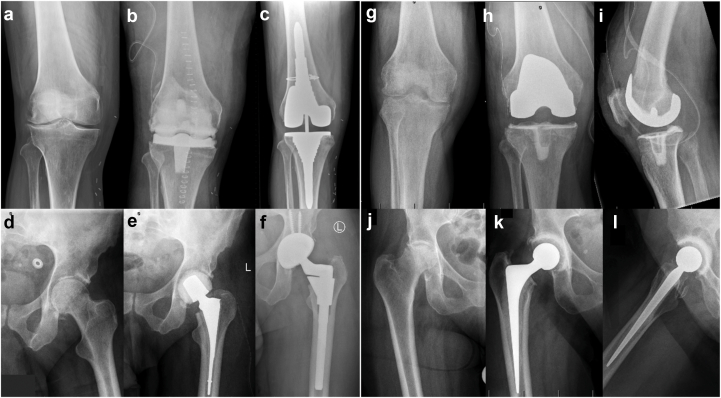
Serial radiographs demonstrating the 2 different treatment approaches for knee and hip NJSA. Two-stage arthroplasty (left): Preoperative images (a, d), postoperative images following spacer implantation (b, e), and final radiographs after second-stage implantation (c, f). 1.5-stage arthroplasty (right): Preoperative images (g, j), postoperative anteroposterior views (h, k), and lateral views (i, l) showing the definitive implant.
